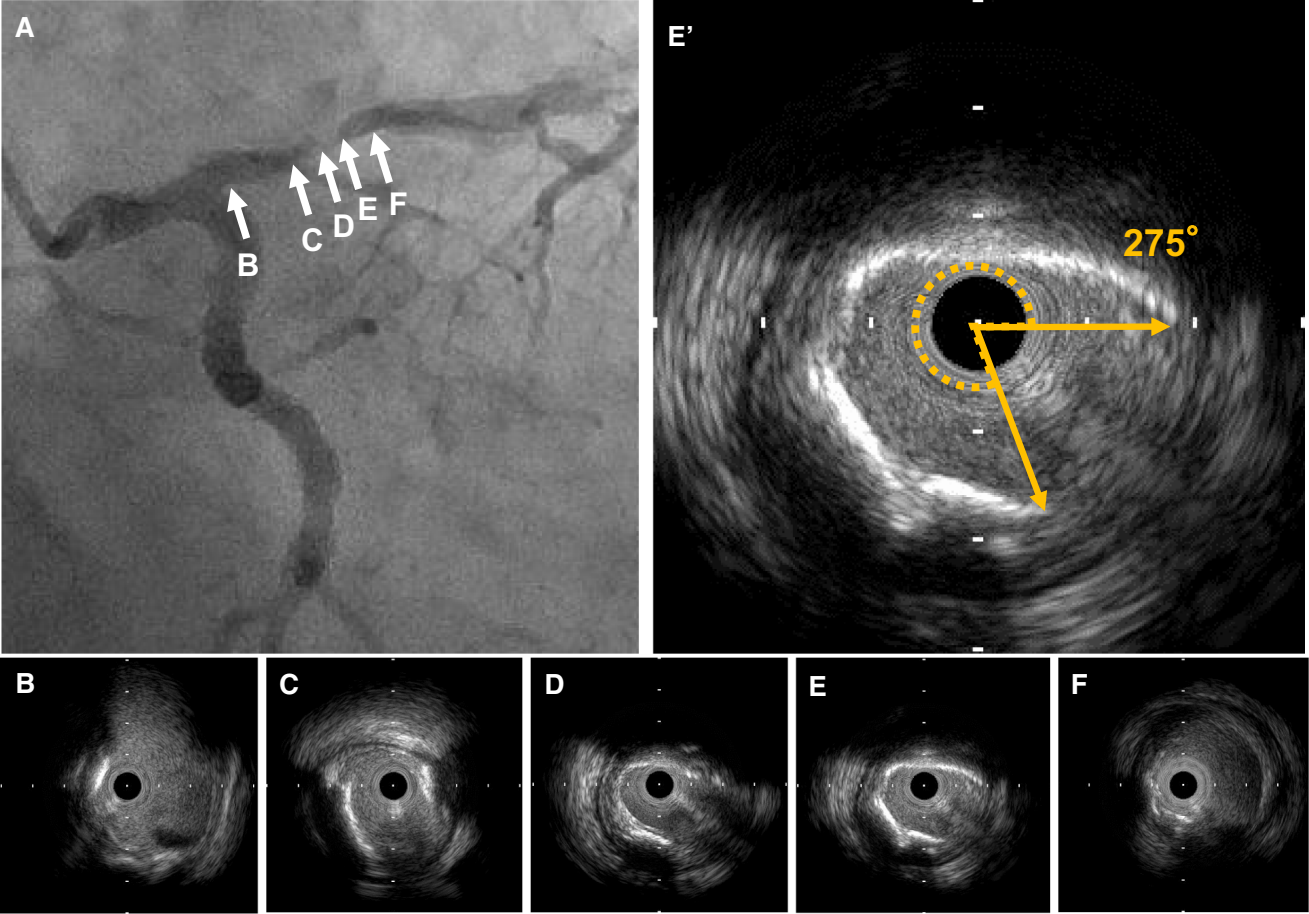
Representative case of intravascular ultrasound-detected attenuated plaque. A 70-year-old man with stable coronary artery disease is shown to have severe coronary artery stenosis on angiography (A). Intravascular ultrasound examination B–F shows a severely calcified plaque (maximum calcification angle, 275°) in the proximal left descending artery (E). In this case, the serum 1,5-anhydro-d-glucitol level is 8.3 µg/mL
